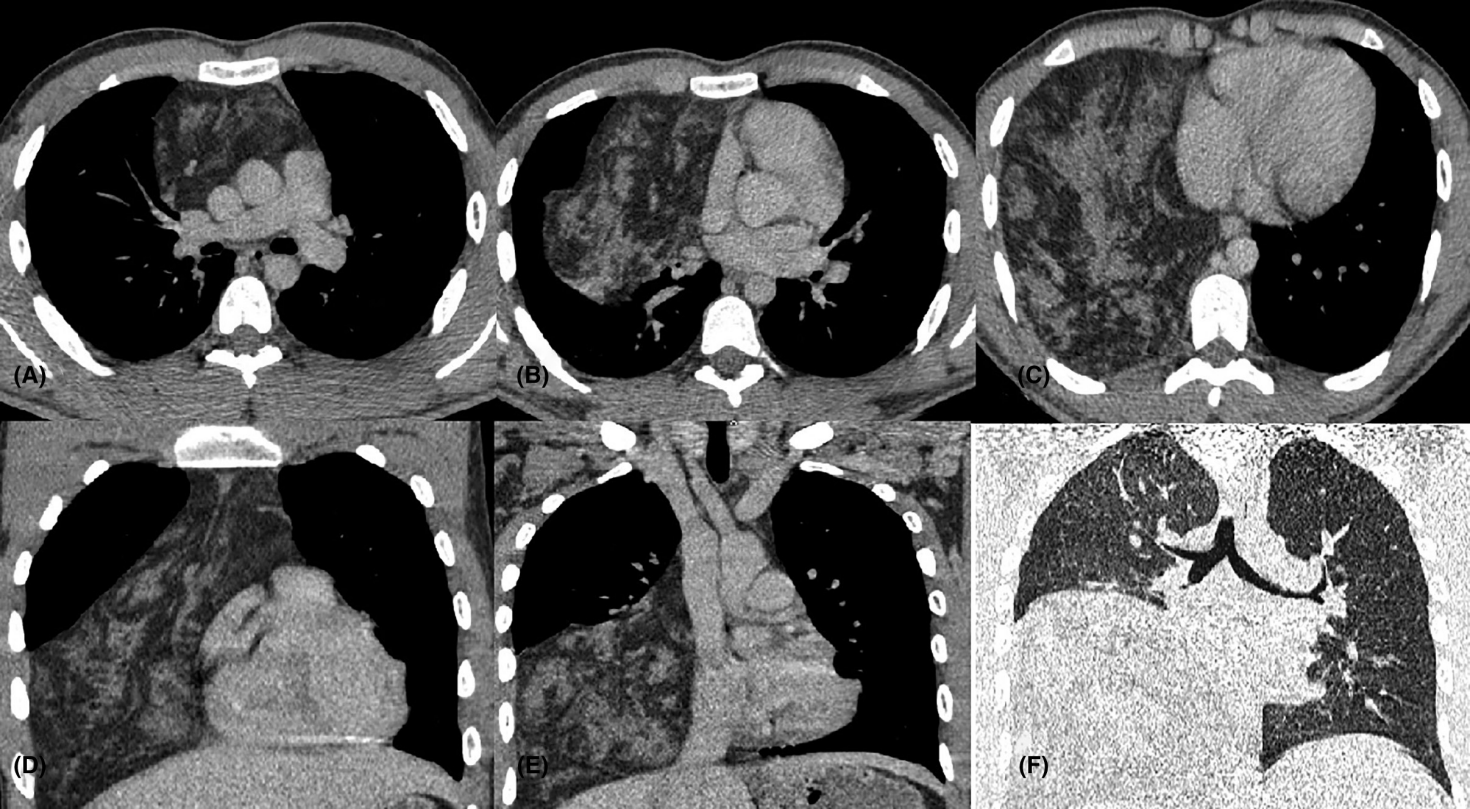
Chest CT scan shows a predominantly fat‐containing mass with soft tissue components in axial (A–C) and the coronal plane (D–E), appearing to originate from the anterior mediastinum and extending into the right hemithorax, causing a shift of the heart and mediastinum to the left. (F) shows near complete collapse of lower lobe of right lung.
